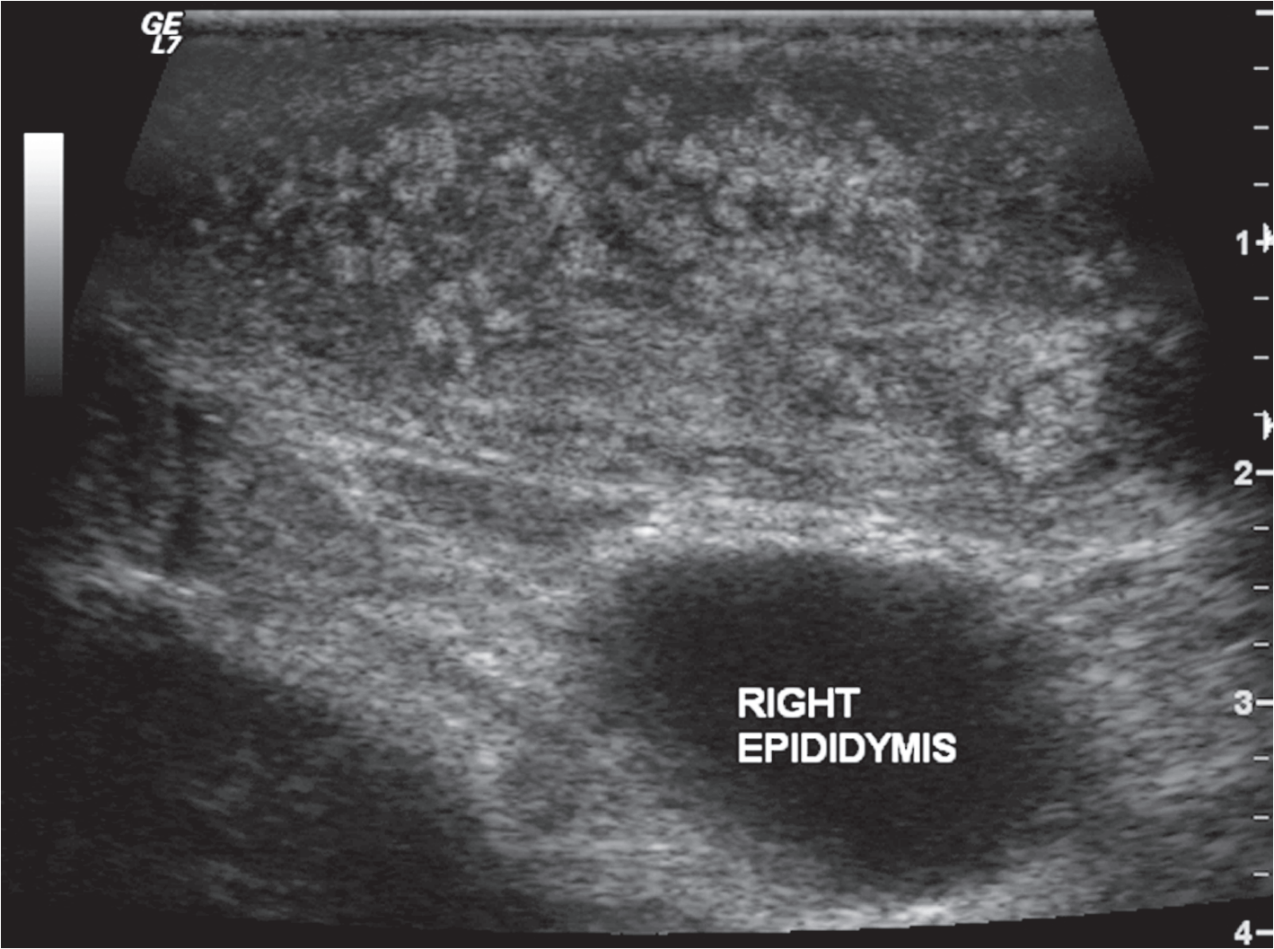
Showing the ultrasound appearance of eumycetoma lesion with numerous grains producing numerous and sharp hyper-reflective echoes. There are multiple thick-walled cavities with no acoustic enhancement.
